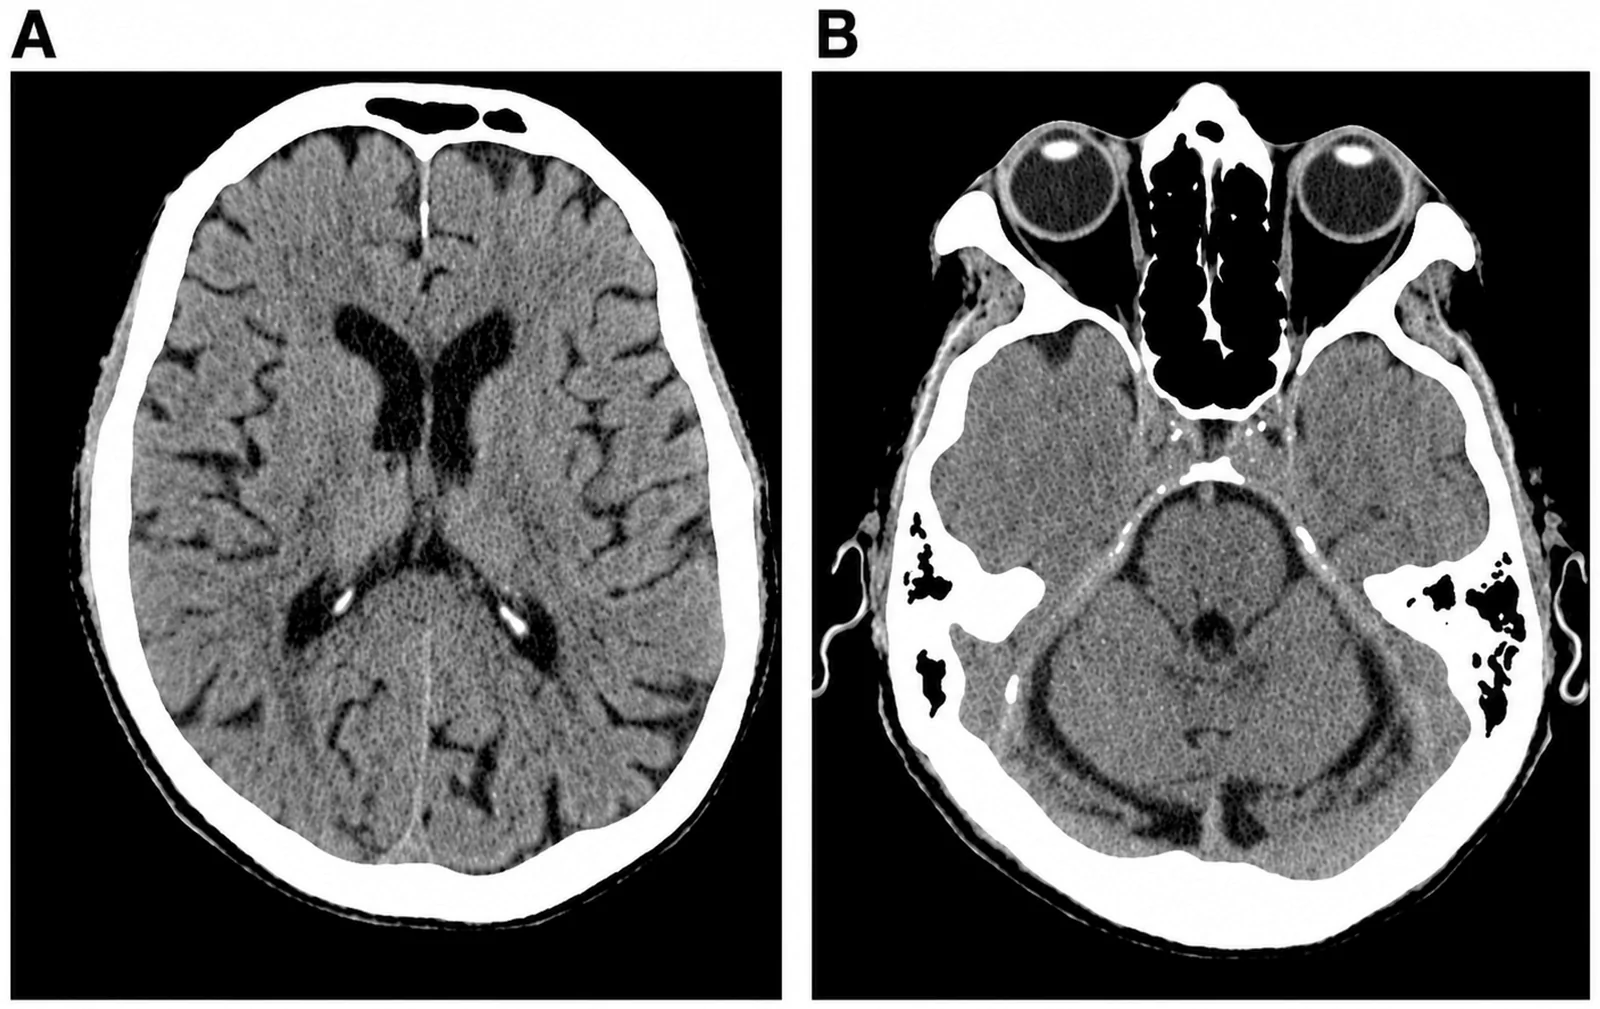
Representative Noncontrast Head Computed Tomography Images Axial noncontrast head computed tomography images obtained after emergency reintubation. (A) Axial image at the level of the lateral ventricles demonstrates preserved ventricular symmetry without acute intracranial hemorrhage, significant mass effect, or midline shift. (B) Axial image through the posterior fossa demonstrates no acute intracranial abnormality. Mild nonspecific white matter changes were reported on the complete examination.
